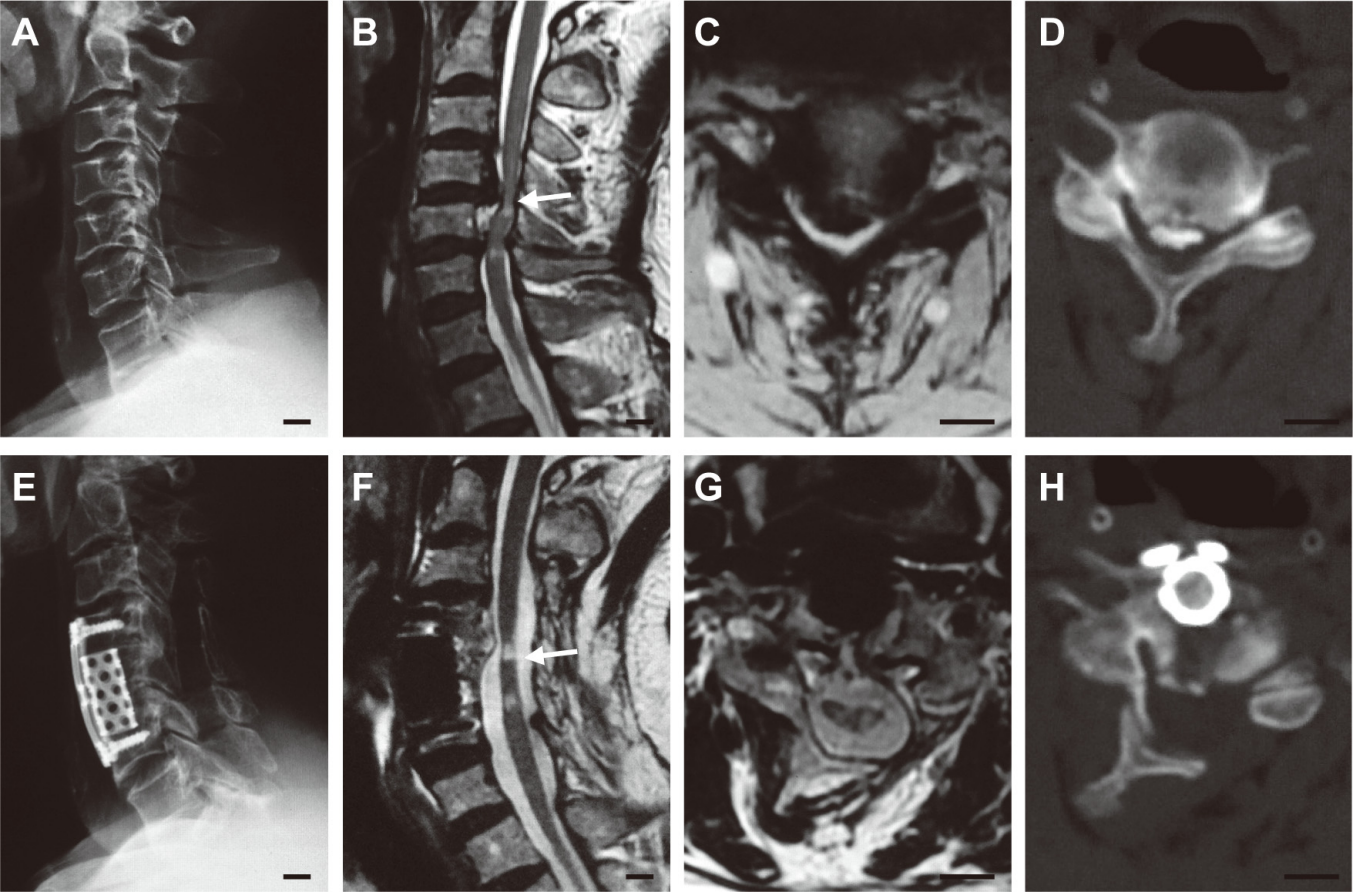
A 53-year-old man who had multilevel cervical spondylotic myelopathy and underwent a combined surgical approach. He suffered from compressions at the C3-6 level (A–D) with a canal occupancy ratio of 54.9% at the maximal compression level (C4-5, compressed by both OPLL and the herniated disc), and was treated with C3-7 laminoplasty followed by C5 ACCF (E–H). Two years after surgery, his JOA score had improved from 8.0 to 16.0 without radiological recompression. (A) Preoperative neutral lateral radiograph. (B) Preoperative T2-weighted sagittal MRI. (C) Preoperative T2-weighted axial MRI at the C4-5 level. (D) Preoperative CT image at the C4-5 level. (E) Postoperative neutral lateral radiograph. (F) Postoperative T2-weighted sagittal MRI. (G) Postoperative T2-weighted axial MRI at the C4-5 level. (H) Postoperative CT image at the C4-5 level. Arrows indicate the maximal compression levels (B, F) where the axial MRI (C, G) and CT (D, H) images should be localized (Scale bar, 10 mm).
